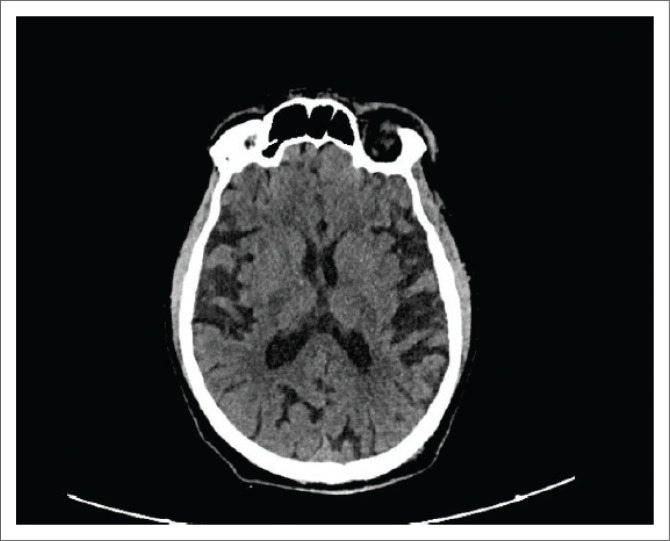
CT scan showing generalised cerebral atrophy.
